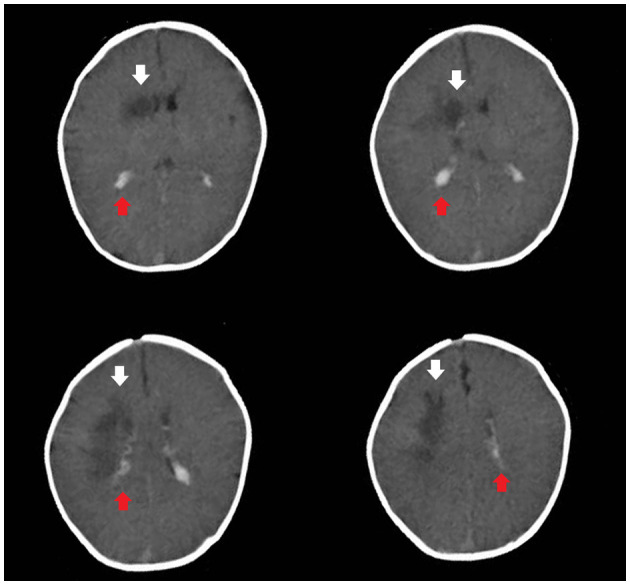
Brain computed tomography revealed subarachnoid hemorrhage (the red arrow) and irregular hypointensity shadows beside the right lateral ventricle (the white arrow).
